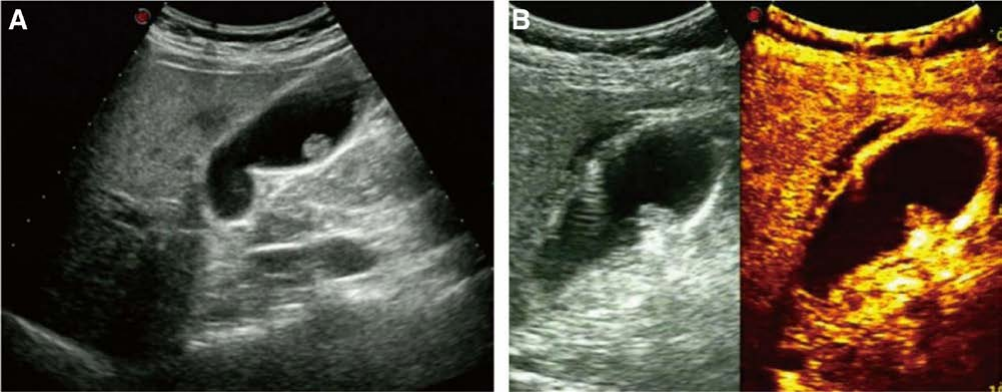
Preoperative ultrasound examination of the patient. (A) Conventional two-dimensional ultrasound showed a medium-high echo papillary eminence on the side wall of the gallbladder, with a wide base and a silent shadow in the back. It did not move when changing position. (B) Preoperative CEUS showed that the lesion was synchronously and uniformly high enhanced with the gallbladder wall in the arterial phase, and the enhancement speed was slightly faster than that of the surrounding liver parenchyma. The attached gallbladder wall was continuous and intact without thickening. CEUS = contrast-enhanced ultrasound.
